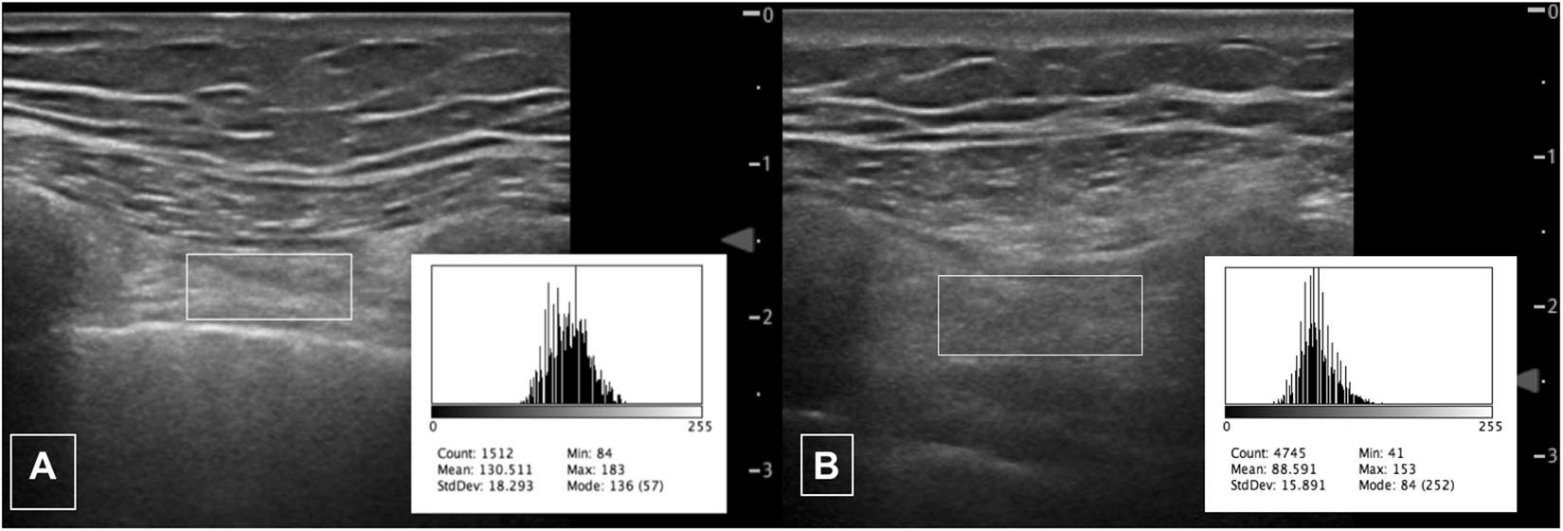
Echogenicity of parasternal intercostal muscle. Typical ultrasound images of parasternal intercostal muscle (right 3^rd^ intercostal muscle) with square to measure region of interest for quantitative grayscale analysis showing (A) higher echogenicity and (B) lower echogenicity. Insets depict respective histograms of grayscale analysis (range 0 [black] to 255 [white]) with mean value recorded.
